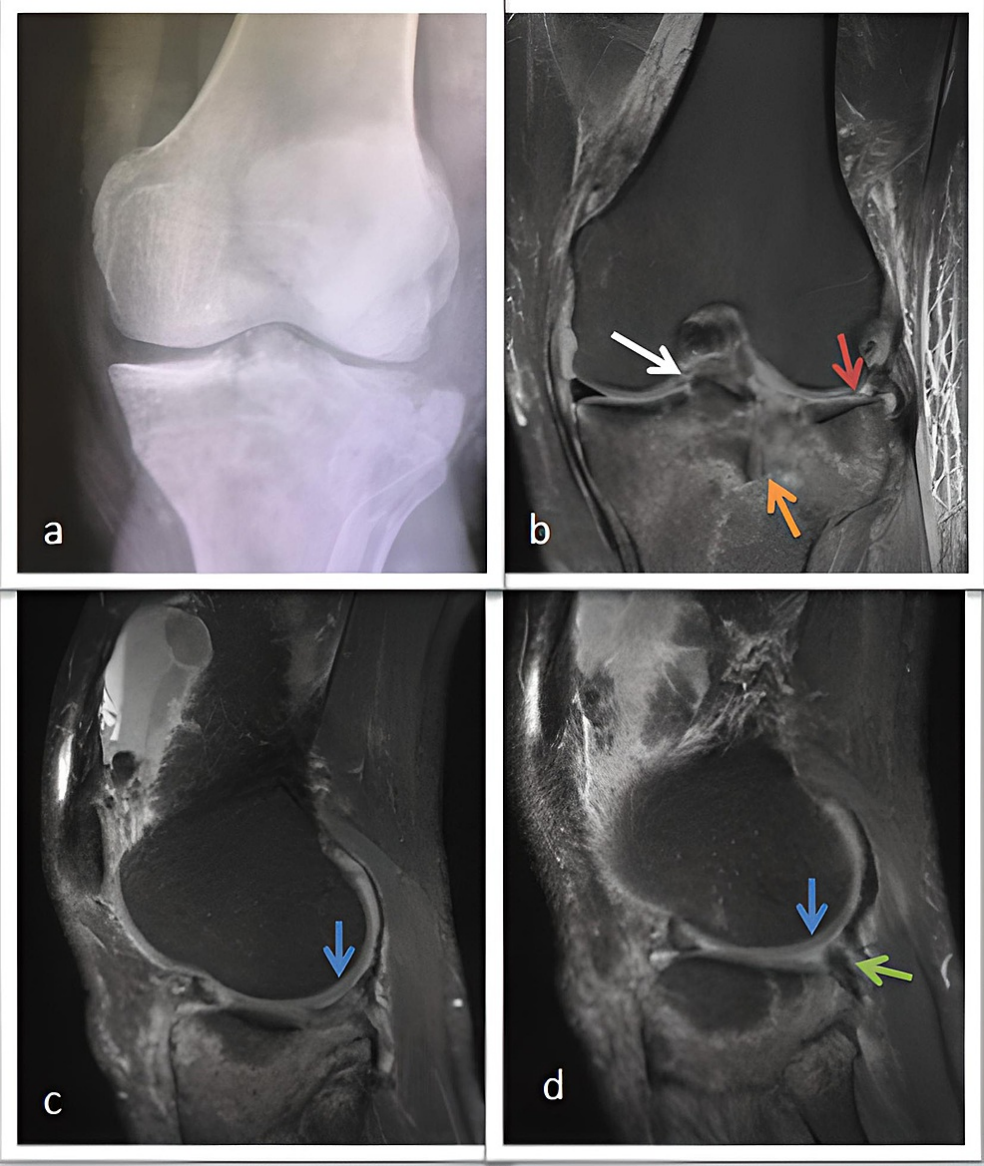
A 43-year-old male patient with a type 2Al fracture according to the novel modified classification. (a) X-ray of type 2 tibial plateau fracture according to the Schatzker classification. (b) FS-PD coronal MRI shows the absence of the corpus of the lateral meniscus (red arrow) due to bucket-handle tear. The white arrow shows the footprint bone avulsion of the ACL, and the orange arrow shows the displaced osteochondral joint surface (c-d) FS-PD sagittal MRI shows the bucket-handle tear of lateral meniscus, the blue arrow shows the absence of the posterior horn of the lateral meniscus, and the green arrow shows the popliteus tendon. FS/PD: fat-suppressed/proton density; ACL: anterior cruciate ligament; MRI: magnetic resonance imaging
